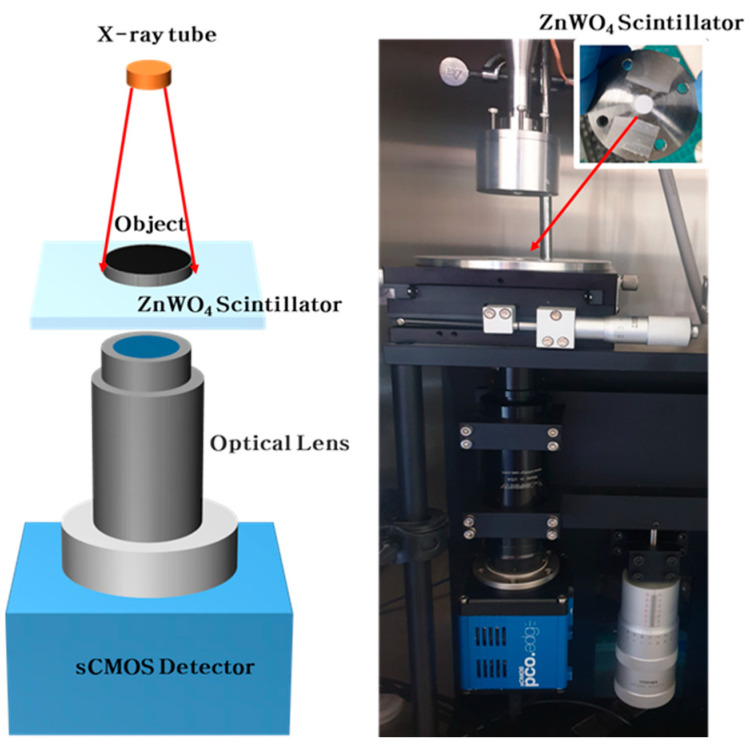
Applying ZnWO_4_ scintillator screen to high-resolution X-ray imaging system.
